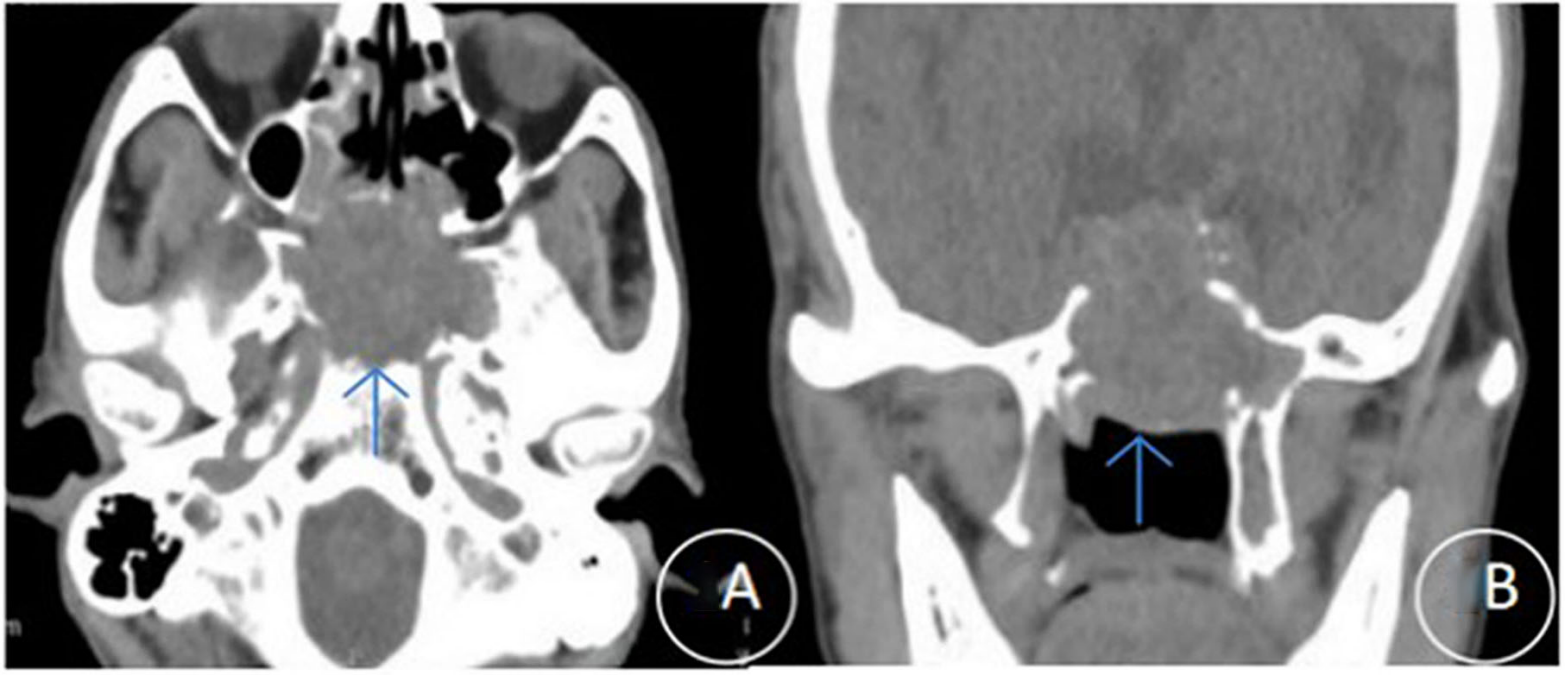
Preoperative CT characteristics of a representative sphenoid sinus tumor. (A, B) CT scans (A) Axial and (B) coronal views show a sphenoid sinus mass (arrows) with associated bone destruction of the clivus and skull base.
